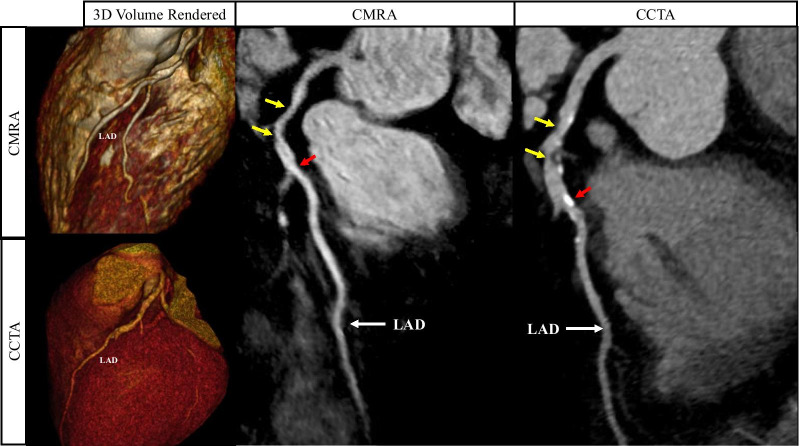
Curved multiplanar reformat and 3D volume rendered non-contrast coronary CMRA and contrast enhanced coronary CTA in a 60 year old male with > 50% partially calcified stenosis in the proximal to mid LAD either side of the first diagonal artery (yellow arrows). The red arrows represent a focal calcified < 50% stenosis just distal to the second diagonal artery
